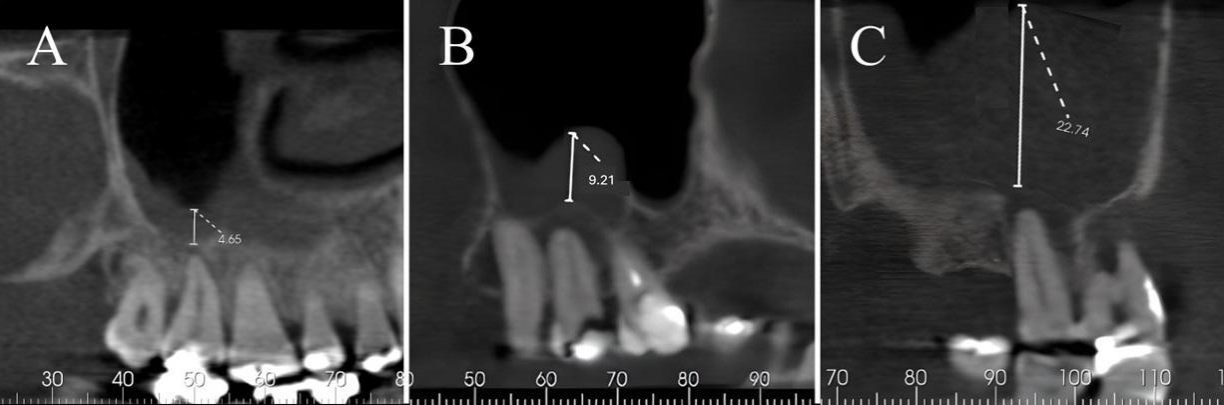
Sagittal section of cone beam tomography images showing the measurements of sinus diseases. A) Thickening of the sinus mucosa > 3mm. B) Partial sinus veiling > 6mm. C) Total sinus veiling > 12mm.
